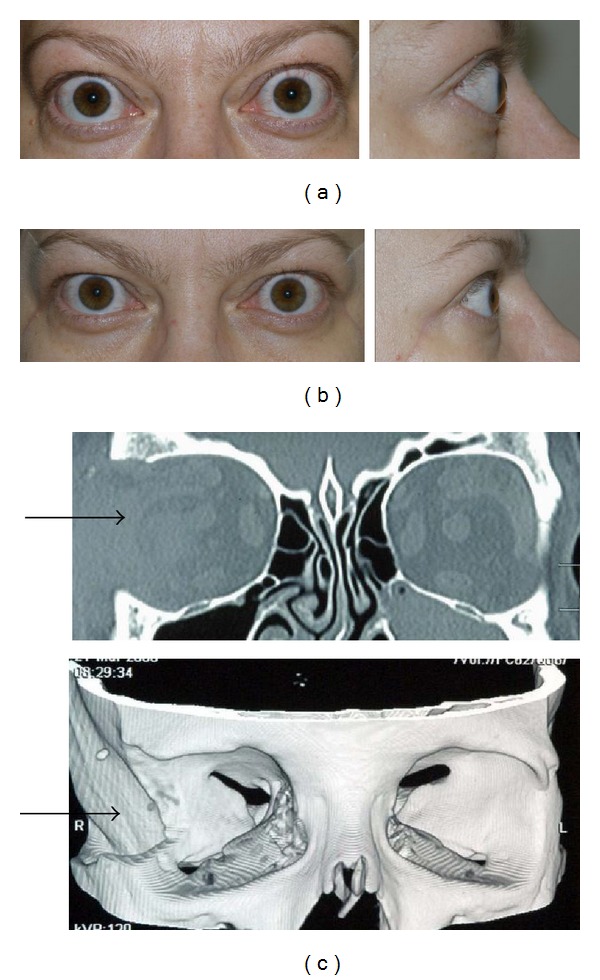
38-year-old female patient with significant exophthalmos and retrobulbar pressure sensation, no visual impairment. (a) Preoperatively: exophthalmos and lid retraction. (b) Postoperatively after lateral wall decompression: exophthalmos improved 5.0 mm on the right side and 4.0 mm on the left side. Additional lid surgery is needed (upper lid lengthening procedure). (c) Postoperatively: CT scan after lateral wall decompression of the right orbit (arrow: missing lateral orbital wall), coronal scan, and 3D reconstruction.
